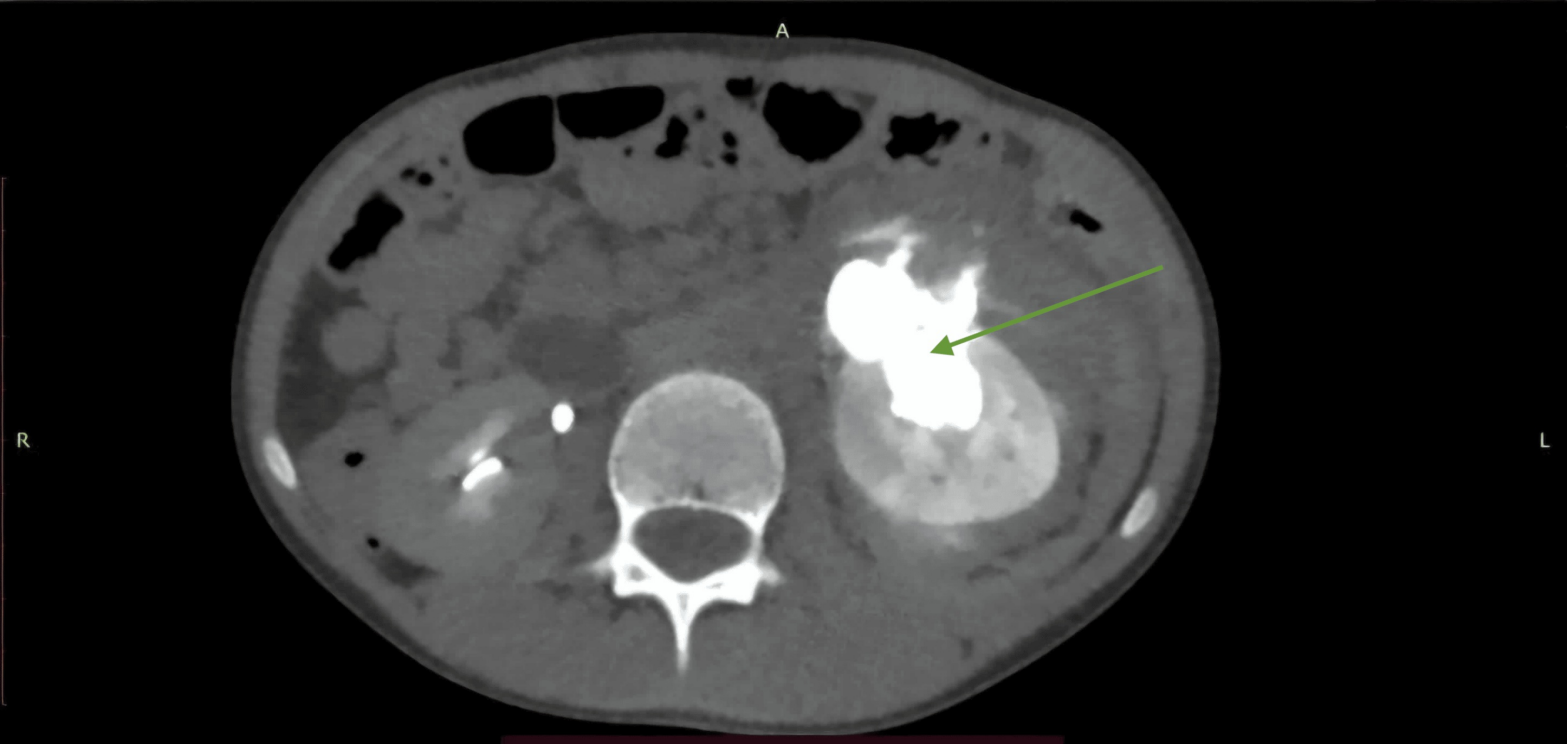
AAST grade IV renal injury with collecting system laceration and contrast extravasation on delayed CECT. A delayed scan in the same patient reveals contrast extravasation (black arrow) from the renal pelvis, indicating a laceration of the collecting system. Findings are consistent with the AAST grade IV renal injury. AAST: American Association for the Surgery of Trauma; CECT: contrast-enhanced computed tomography.
